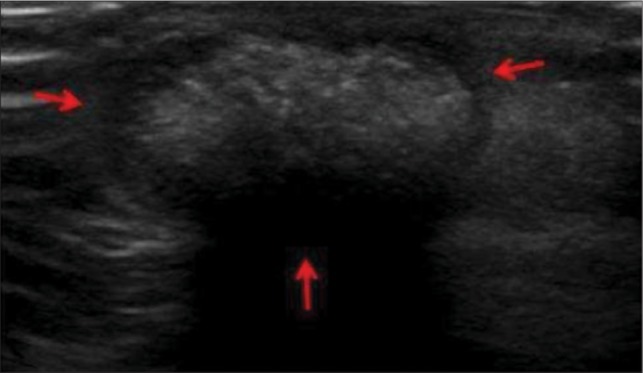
A 13-year-old boy with pilomatricoma who presented with dorsum of hand swelling. (a) Gray scale ultrasound image reveals superficial solid mass with typical hypoechoic rim (red arrows) and internal echogenic calcifications.
